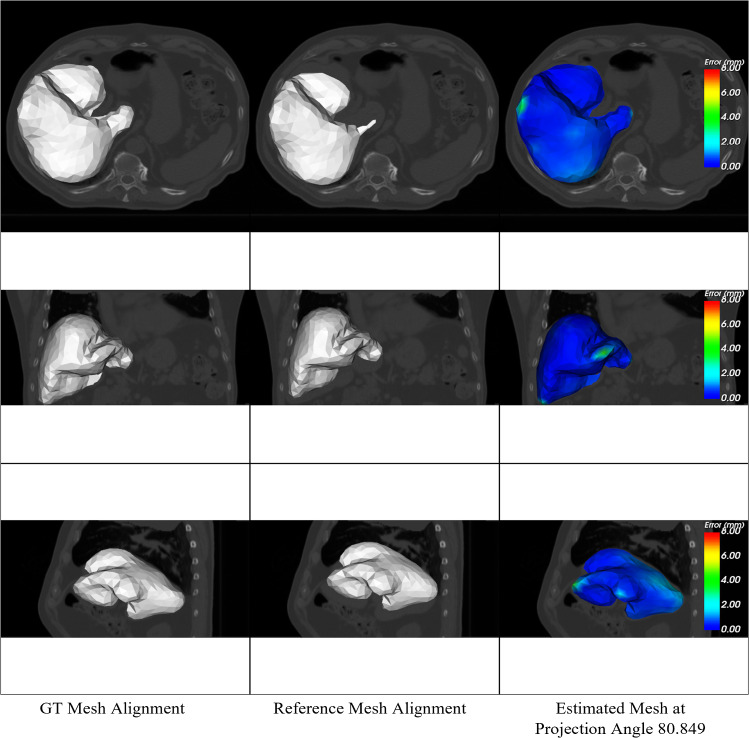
Visualisations of ground-truth deformed (left), template (middle), and estimated deformed (right) 3D liver shapes overlaid on the deformed 3D CT volume. Rows 1-3 show axial, coronal, and sagittal views, respectively. Results are for the *worst* performing test case for patient 1: projection angle $$80.849^\circ $$ and deformation state with the highest errors. Contours in the right column show the spatial error distribution on the surface. Results for patients 2–4 are in Sect. Discussion of Online Resource 1
